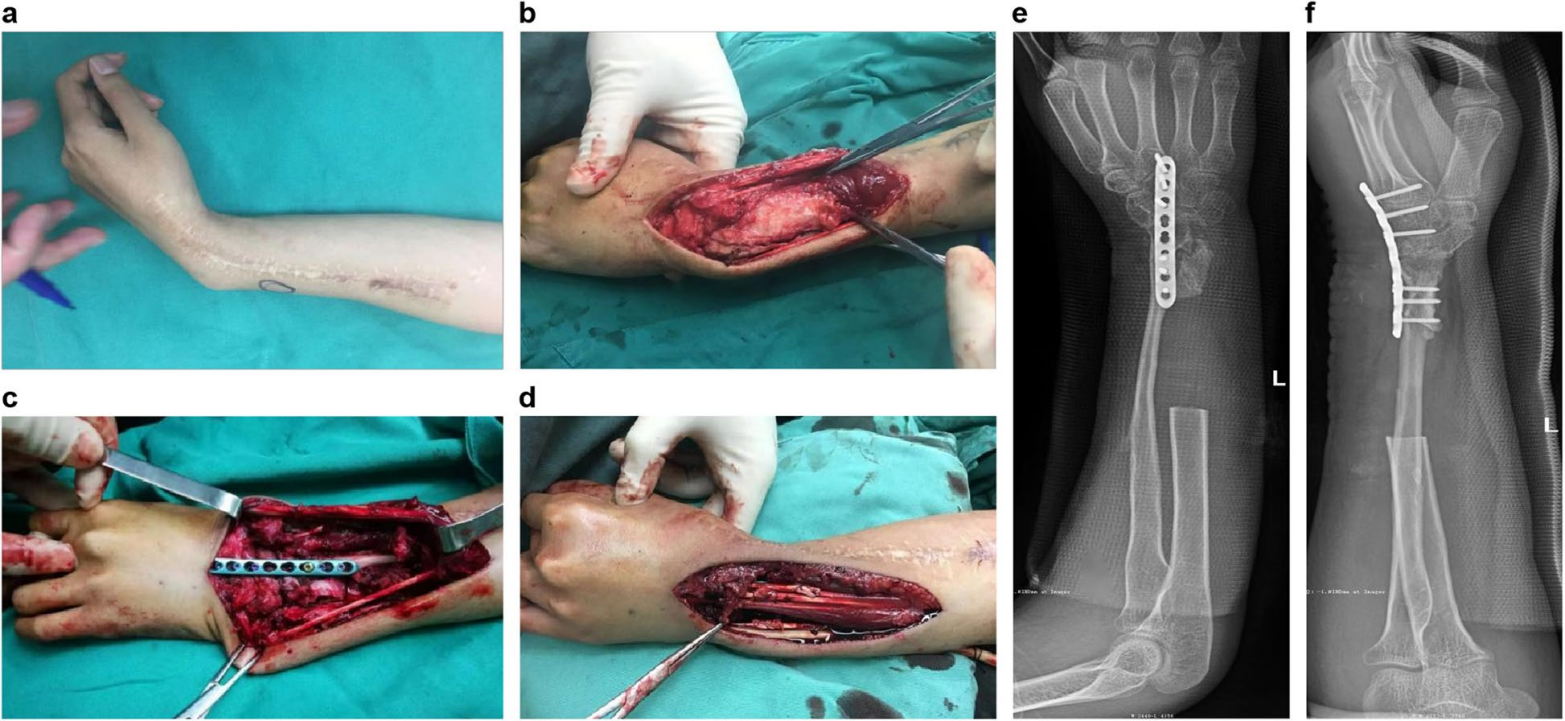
a The patient underwent a second tumor resection; b an irregular mass with cortical breakthrough is observed; c tumor resection and wrist fusion were performed in a single stage; d the extensor tendons were cut off and repaired at different levels. e Anteroposterior and f lateral radiographs showing approximately 10 cm length of the resected affected ulna, the corrected wrist deformity, and a 2.7 mm dynamic compression plate used for wrist fusion
